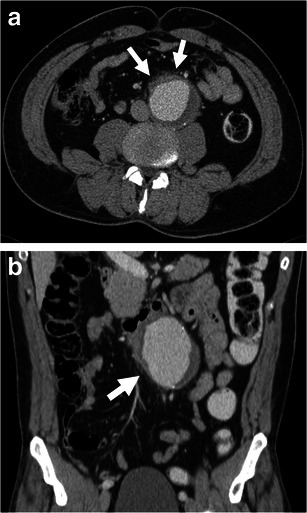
Periaortic stranding. a Axial and b coronal enhanced CT of a 51-year-old man with abdominal pain shows stranding of periaortic fat (*white arrows*) before any retroperitoneal haematoma can be seen
